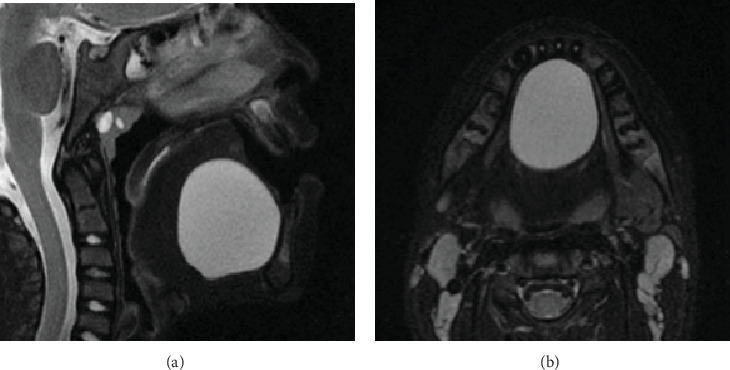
T2-weighted magnetic resonance imaging before the cyst removal. (a) Sagittal view. (b) Axial view.
